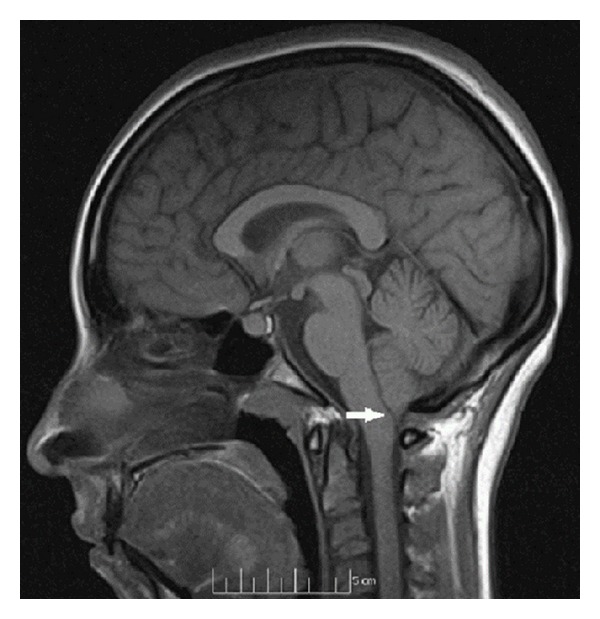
Sagittal magnetic resonance image of Arnold-Chiari type I malformation. White arrow denotes the 7 mm tonsillar herniation from the cerebellum. No syringomyelia is seen.
